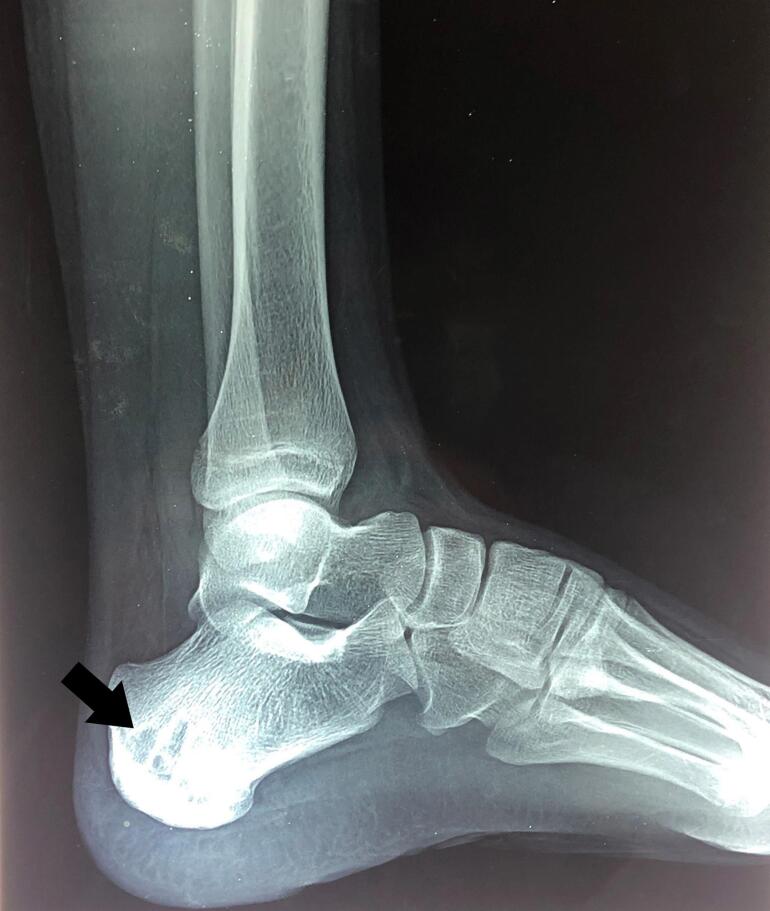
Lateral radiograph of left foot showing an osteolytic lesion in the calcaneum (arrow).
